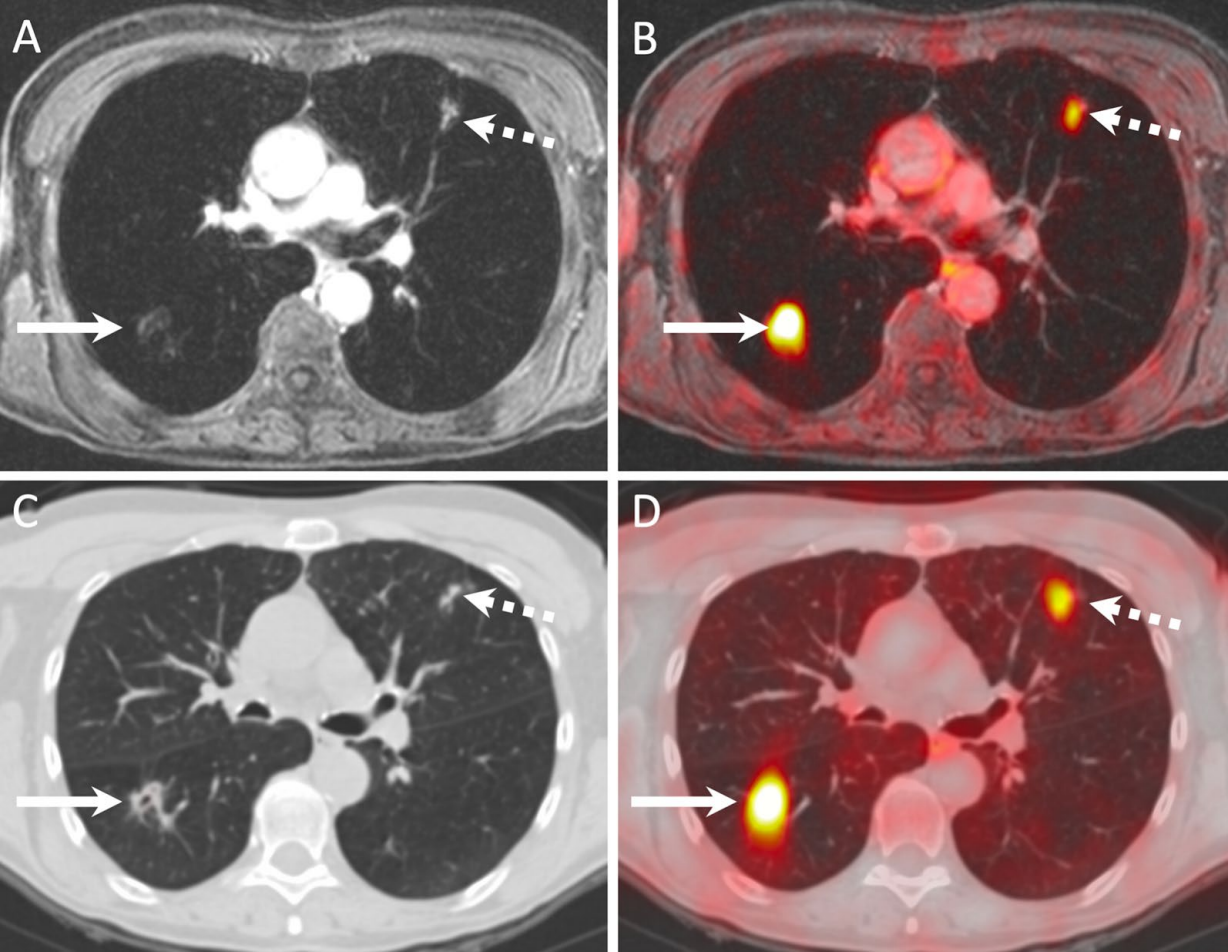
Lung metastases correctly diagnosed on PET/MRI (A, B) and PET/CT (C, D) in a 60-year-old female with nodal recurrence after radiochemotherapy for oropharyngeal HPV-negative SCC. The right upper lobe metastasis (arrows) shows a combination of high focal FDG uptake (SUVmax on PET/MRI = 7.7 and SUVmax on PET/CT = 9.3) and an excavated aspect on the contrast-enhanced fat-saturated MR image and on the corresponding CT image. The left upper lobe metastasis (dashed arrows) displays minor focal [^18^F]FDG uptake (SUVmax on PET/MRI = 1.7 and SUVmax on PET/CT = 2.1) and clustered nodules on the corresponding morphologic MRI/CT images. Both lesions were rated with a score of 5 on PET/MRI and PET/CT
